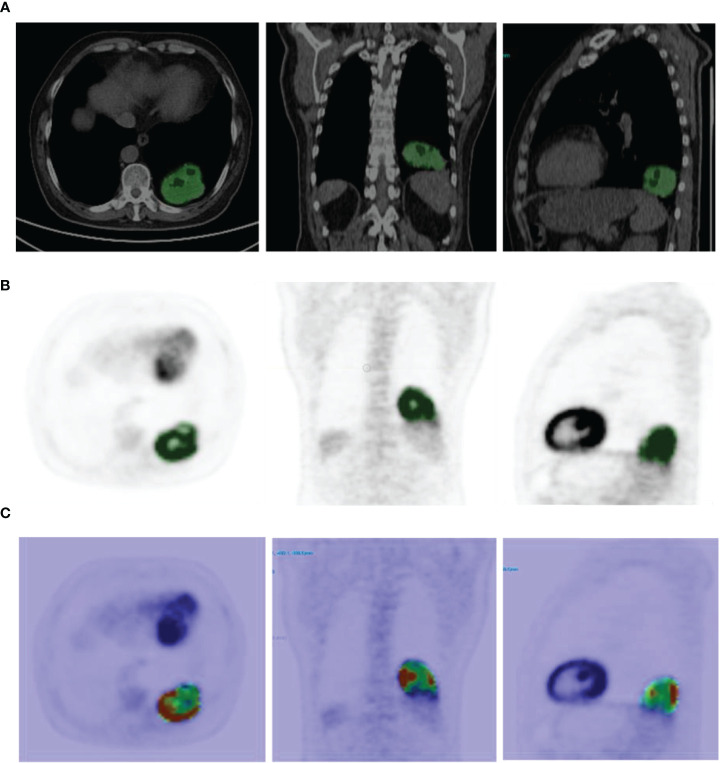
(A) Example of CT image segmentation (the green area is the VOI drawn, the same as below). (B) Example of ^68^Ga-NOTA-PRGD2 PET image segmentation. (C) Example of feature map extracted (GLCM Dissimilarity).
